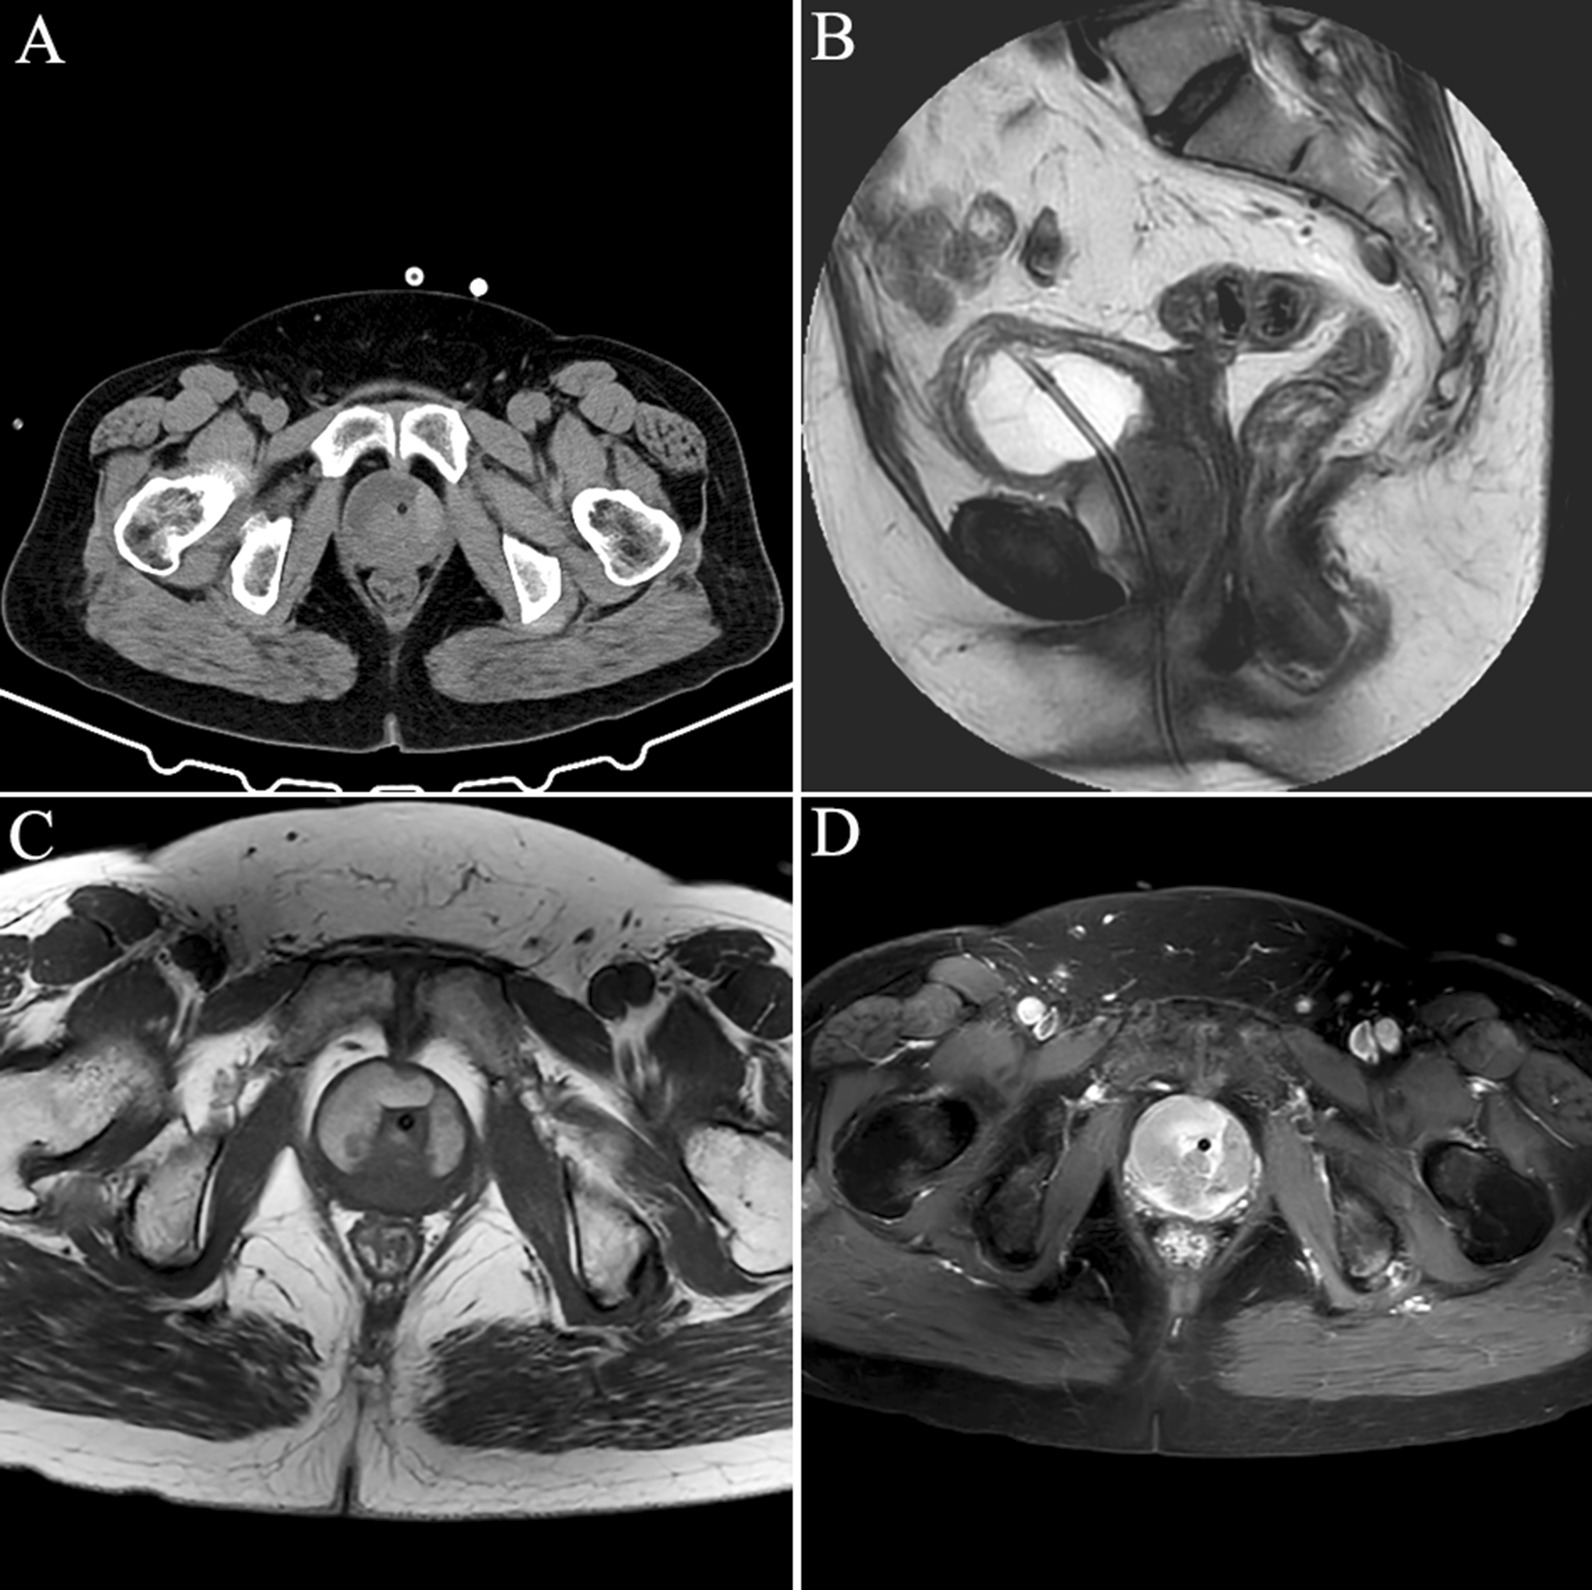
The CT and MRI features of the periurethral mass. a Pelvic CT scan; b sagittal T2-weighted MRI image; c axial T1-weighted MRI image; d axial T1 image with contrast
